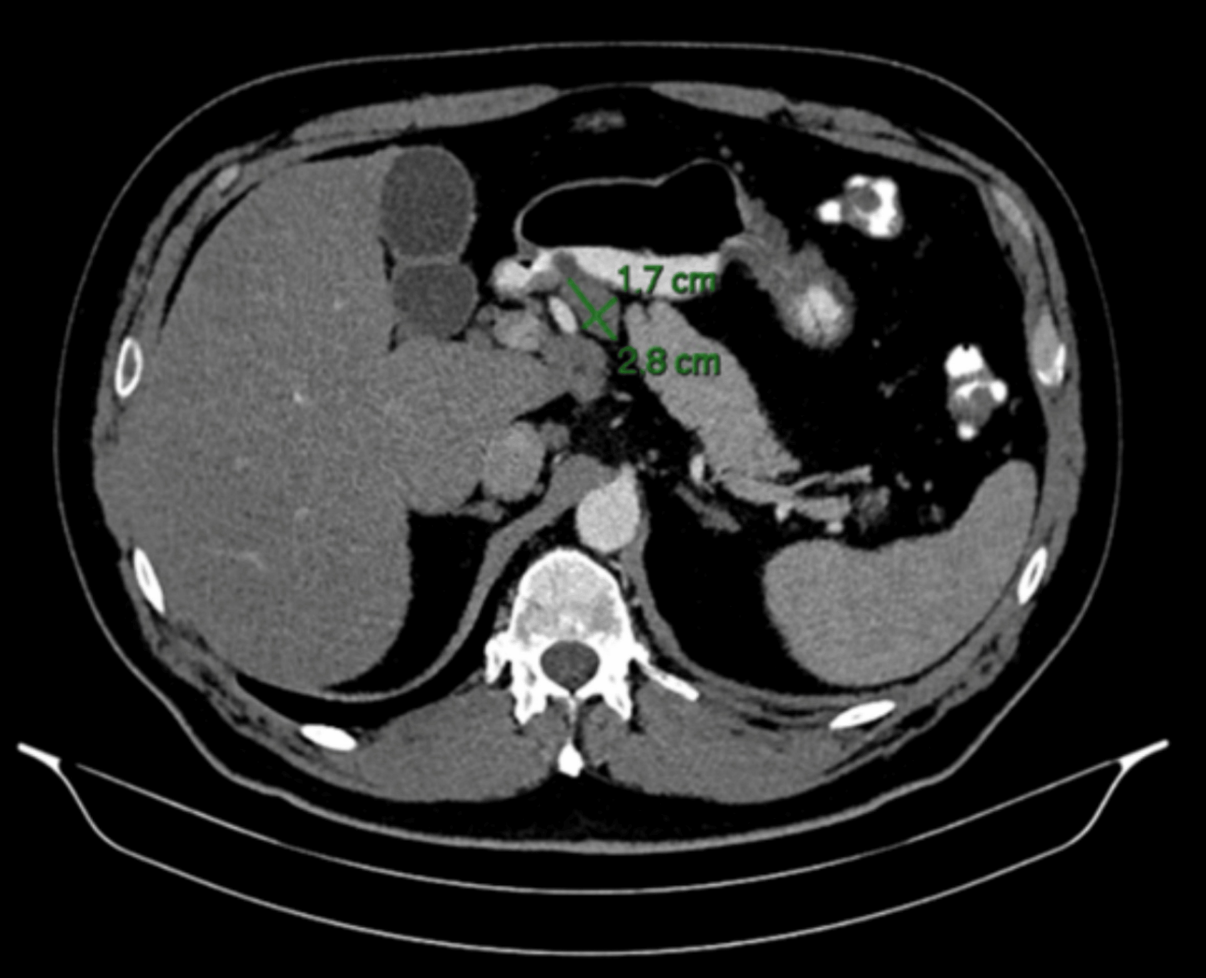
CT abdomen (axial view), showing second prominent lymph node adjacent to the gastric antrum (2.8 × 1.7 cm)
